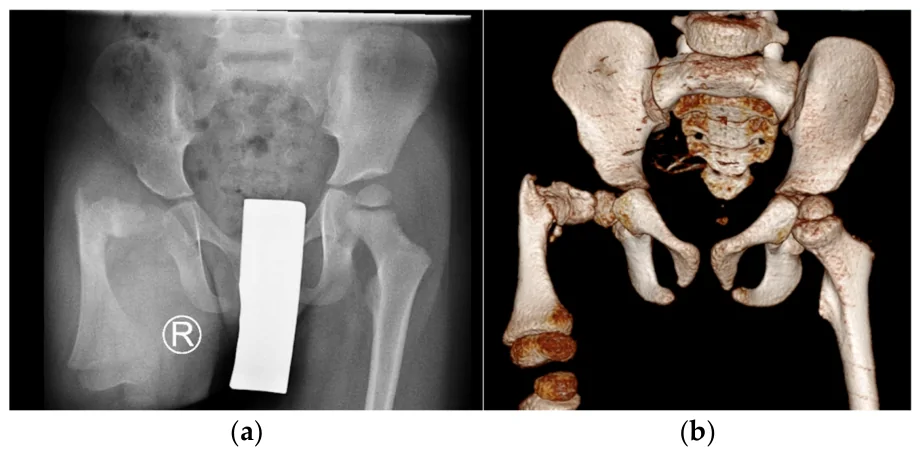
Imaging studies of a 5-year-old patient with right-sided CFD type 1B combined type (1B3): (a) radiograph; (b) 3D CT reconstruction. R—right.
